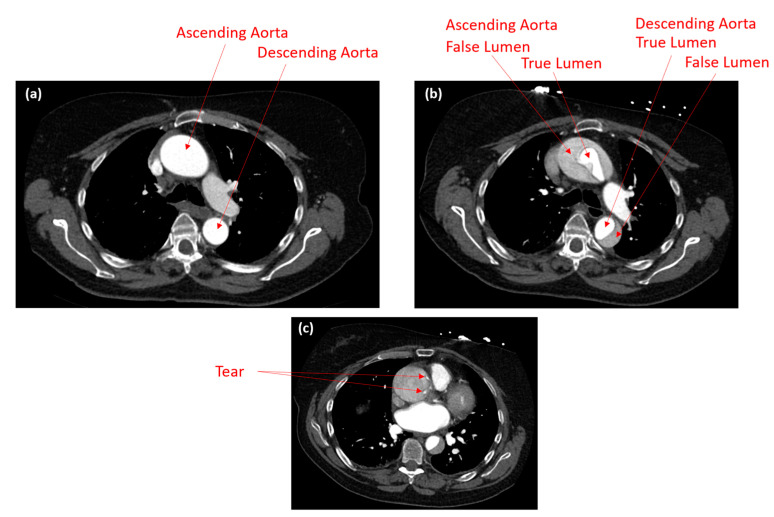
(a) The CT scan for the before AD event showing the ascending and descending aorta; (b) the CT scan showing the post-AD event when the dissection occurred at the ascending and descending aorta showing the false and true lumen; (c) the location of the tear for the Type A AD.
